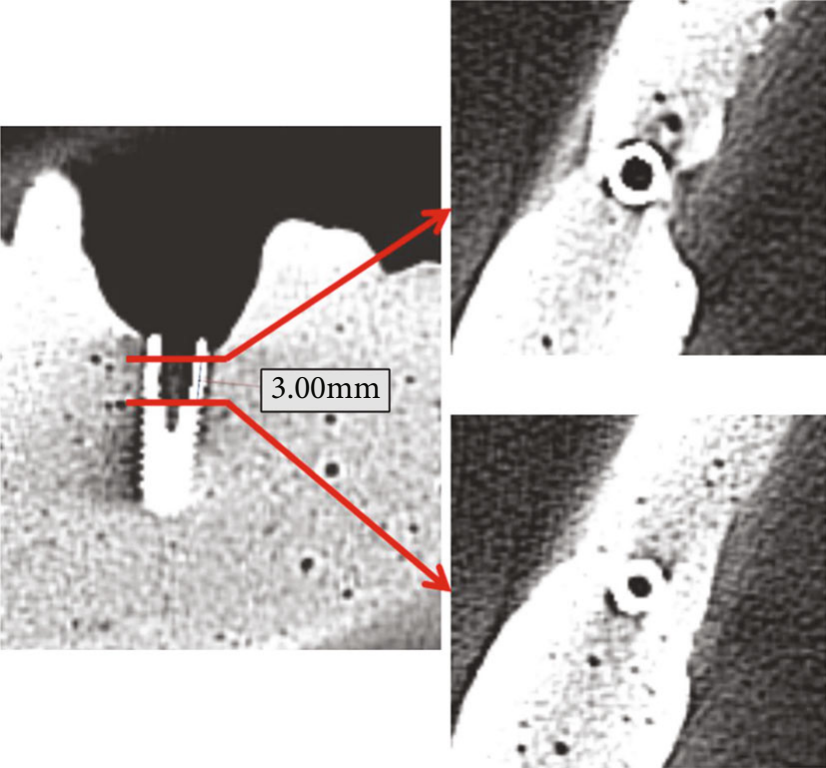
Selection of axial sections from CBCT images.
